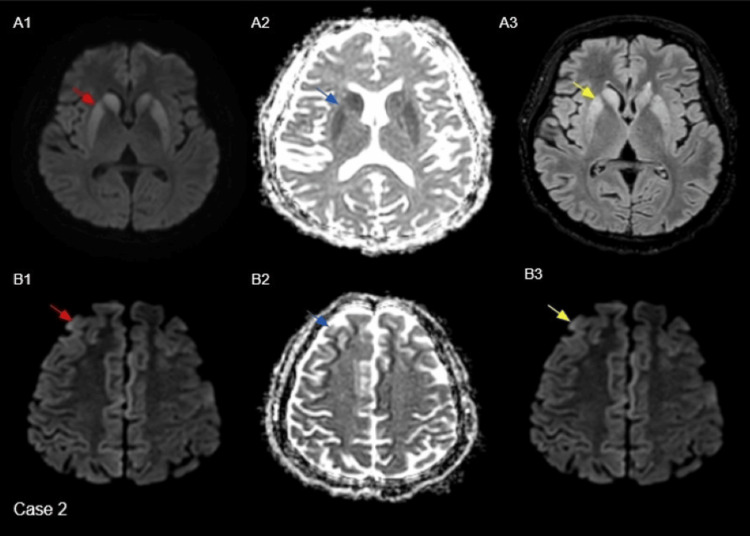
Initial MRI: Axial B-1000 diffusion images (red arrows in A1) show diffusion hyperintensity involving the bilateral striatum, demonstrating an anteroposterior gradient. Corresponding ADC maps (blue arrows in A2) reveal reduced signal in the same regions, and FLAIR images (yellow arrows in A3) show matching hyperintensity. Follow-up MRI after 20 days: Axial B-1000 diffusion images (red arrows in B1) demonstrate new cortical hyperintensity involving the bilateral frontal lobes. Corresponding ADC maps (blue arrows in B2) show reduced signal, and FLAIR images (yellow arrows in B3) reveal associated hyperintensity. The thalamus and cerebellum appear normal. ADC: apparent diffusion coefficient; FLAIR: fluid-attenuated inversion recovery
